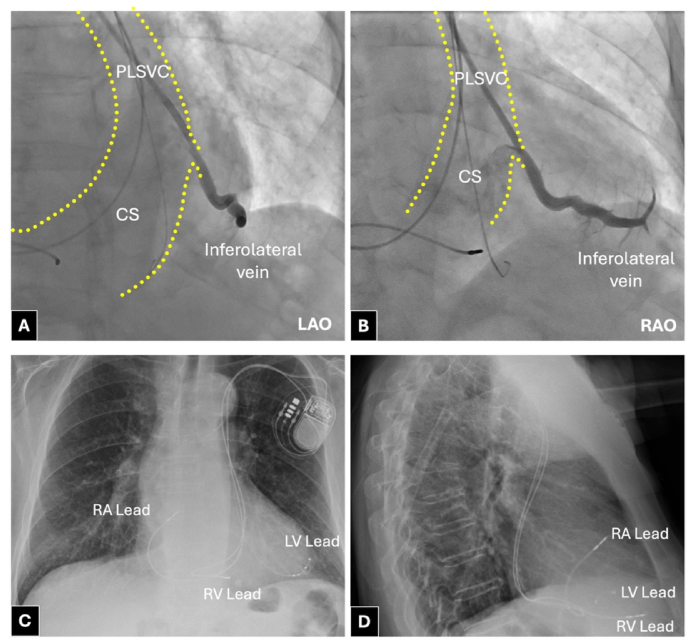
Cardiac resynchronization therapy implantation in a patient with persistent left superior vena cava (PLSVC). (A) Left anterior oblique (LAO) projection: selective venography of the inferolateral vein demonstrating the characteristic tortuous course of the delivery system through the dilated coronary sinus - a hallmark of PLSVC anatomy. (B) Right anterior oblique (RAO) projection: selective venography confirming the inferolateral trajectory along the lateral left ventricular wall. (C) Posteroanterior chest radiograph following CRT-P implantation. The left ventricular lead follows the characteristic "double curve" pathway: descending via the PLSVC, coursing through the dilated coronary sinus, and terminating in the inferolateral vein. Note the significant lead redundancy within the CS - an unavoidable consequence of the elongated venous route requiring careful slack management. (D) Lateral chest radiograph confirming final lead positions. The looping of the LV lead within the dilated CS is clearly visible. The relatively apical lead position reflects the limited tributary options often encountered in PLSVC, where mid-ventricular segments may lack adequate vein caliber or stability for reliable lead fixation. Abbreviations: CS, coronary sinus; CRT-P, cardiac resynchronization therapy pacemaker; LV, left ventricular; PLSVC, persistent left superior vena cava; RA, right atrial; RV, right ventricular.
